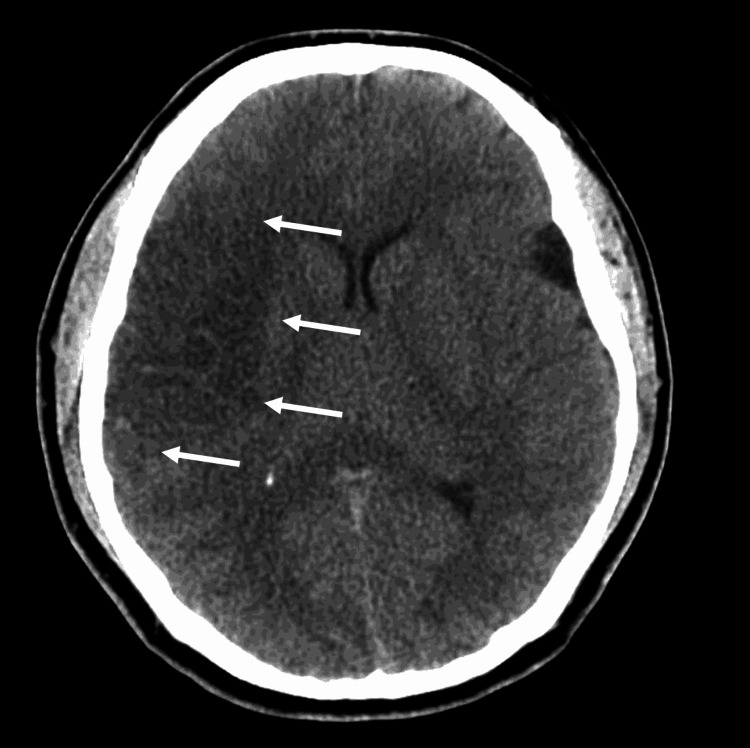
CT of the head revealed a significant right-sided MCA stroke (arrows) as well as a left-sided arachnoid cyst, further evaluated with MRI. CT: computed tomography; MCA: middle cerebral artery; MRI: magnetic resonance imaging
